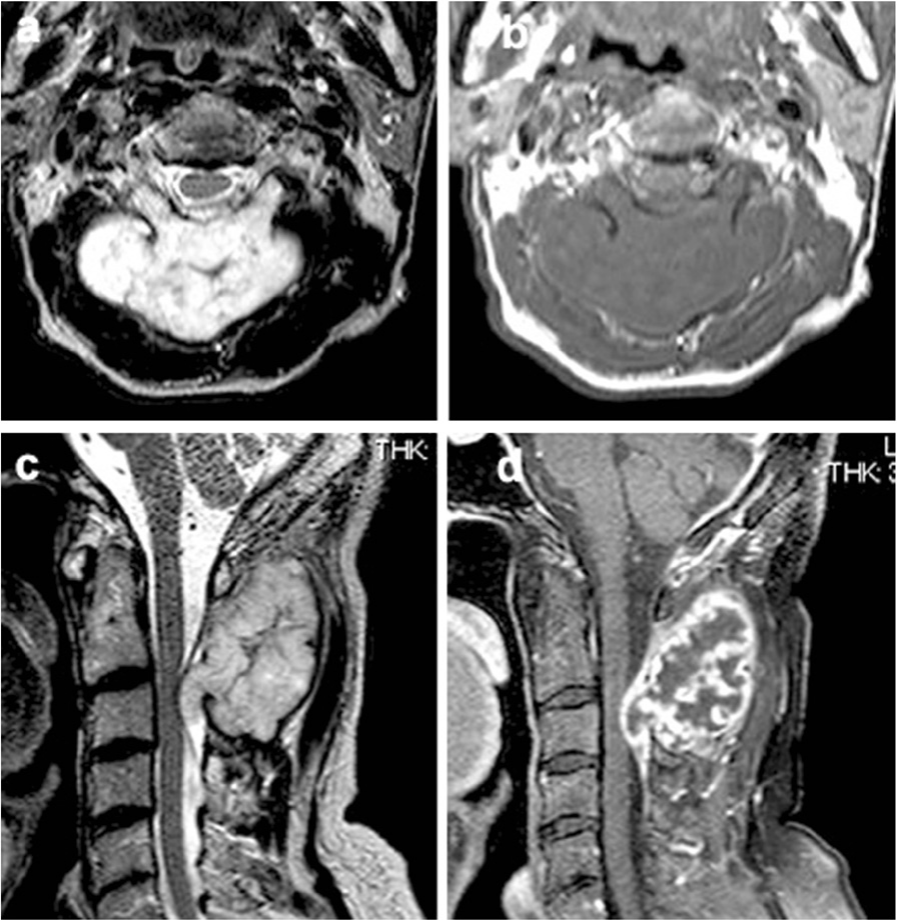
Transverse T2 (a) and T1-weighted images at C2 (b), a sagittal T2-weighted image (c) and T1-weighted image after the intravenous administration of gadolinium (d), of the cervical spine. During the MR imaging, the lesion exhibited a lobulated lesion expanding over the entire lamina and pedicles of C2 with the tumor protuberant to the adjacent soft tissue ((a) and (b)). The mass was low-intensity on T1-weighted images and had heterogeneous low and high-intensities on T2-weighted images, suggesting the presence of mineralized and nonmineralized matrices. Gadolinium-enhanced T1-weighted fat saturated images showed intense peripheral and lobulated rim enhancement, whereas lesions with limited mineralization may appear with homogenous enhancement (d). MRI also showed the epidural extension of the mass at the C2/3 level (c).
